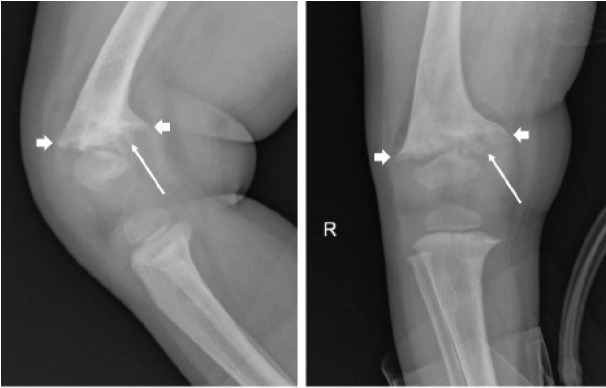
Right knee radiograph of a 19-month-old girl with seizure demonstrating fraying (long arrows) and splaying (short arrows) of the metaphysis.
